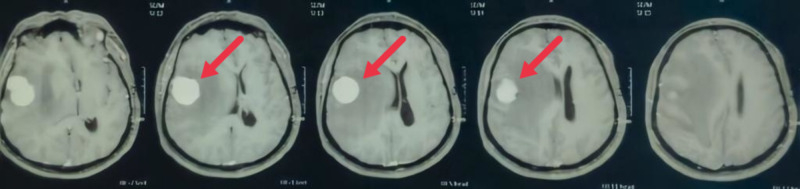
Skull CT with right front-temporo-insular lesion.
